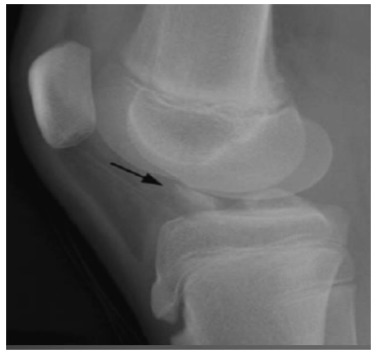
The perfect lateral X-ray to diagnose tibial eminence fracture.
